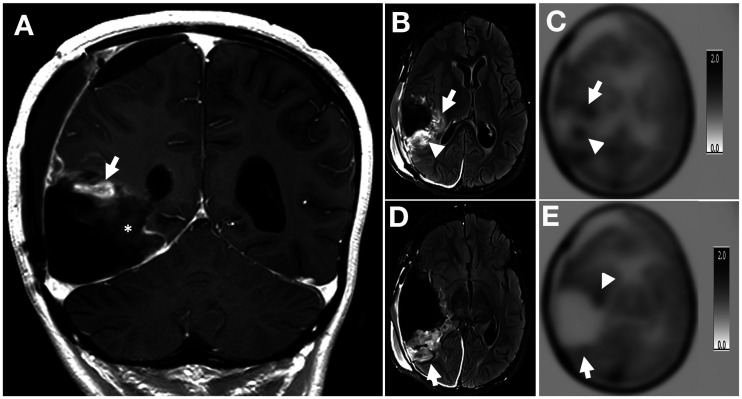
(A) Postcontrast coronal T1-weighted image demonstrates nodular enhancement (arrow) at superior surgical margin. (B) Axial T2-weighted FLAIR image obtained through level of nodular enhancement seen in A demonstrates areas of heterogeneously hyperintense tissue at medial (arrow) and posterior (arrowhead) surgical margin. (C) Axial reconstruction of ^11^C-methionine PET images through this level shows 2 foci of tracer uptake at medial (arrowhead) and posterior (arrow) surgical margin. (D) Axial T2-weighted FLAIR image obtained through plane (demarcated by asterisk in A) inferior to plane of images B and C demonstrates relatively large areas of heterogeneously hyperintense tissue at posterior surgical margin (arrow). (E) Axial reconstruction of ^11^C-methionine PET images through this level shows no ^11^C-methionine uptake at posterior surgical margin (arrow). There is minimum uptake at anteromedial surgical margin (arrowhead). This area was not included in metabolic tumor volume because of low SUV (lower than 1.3 times that of contralateral frontal lobe cortex).
